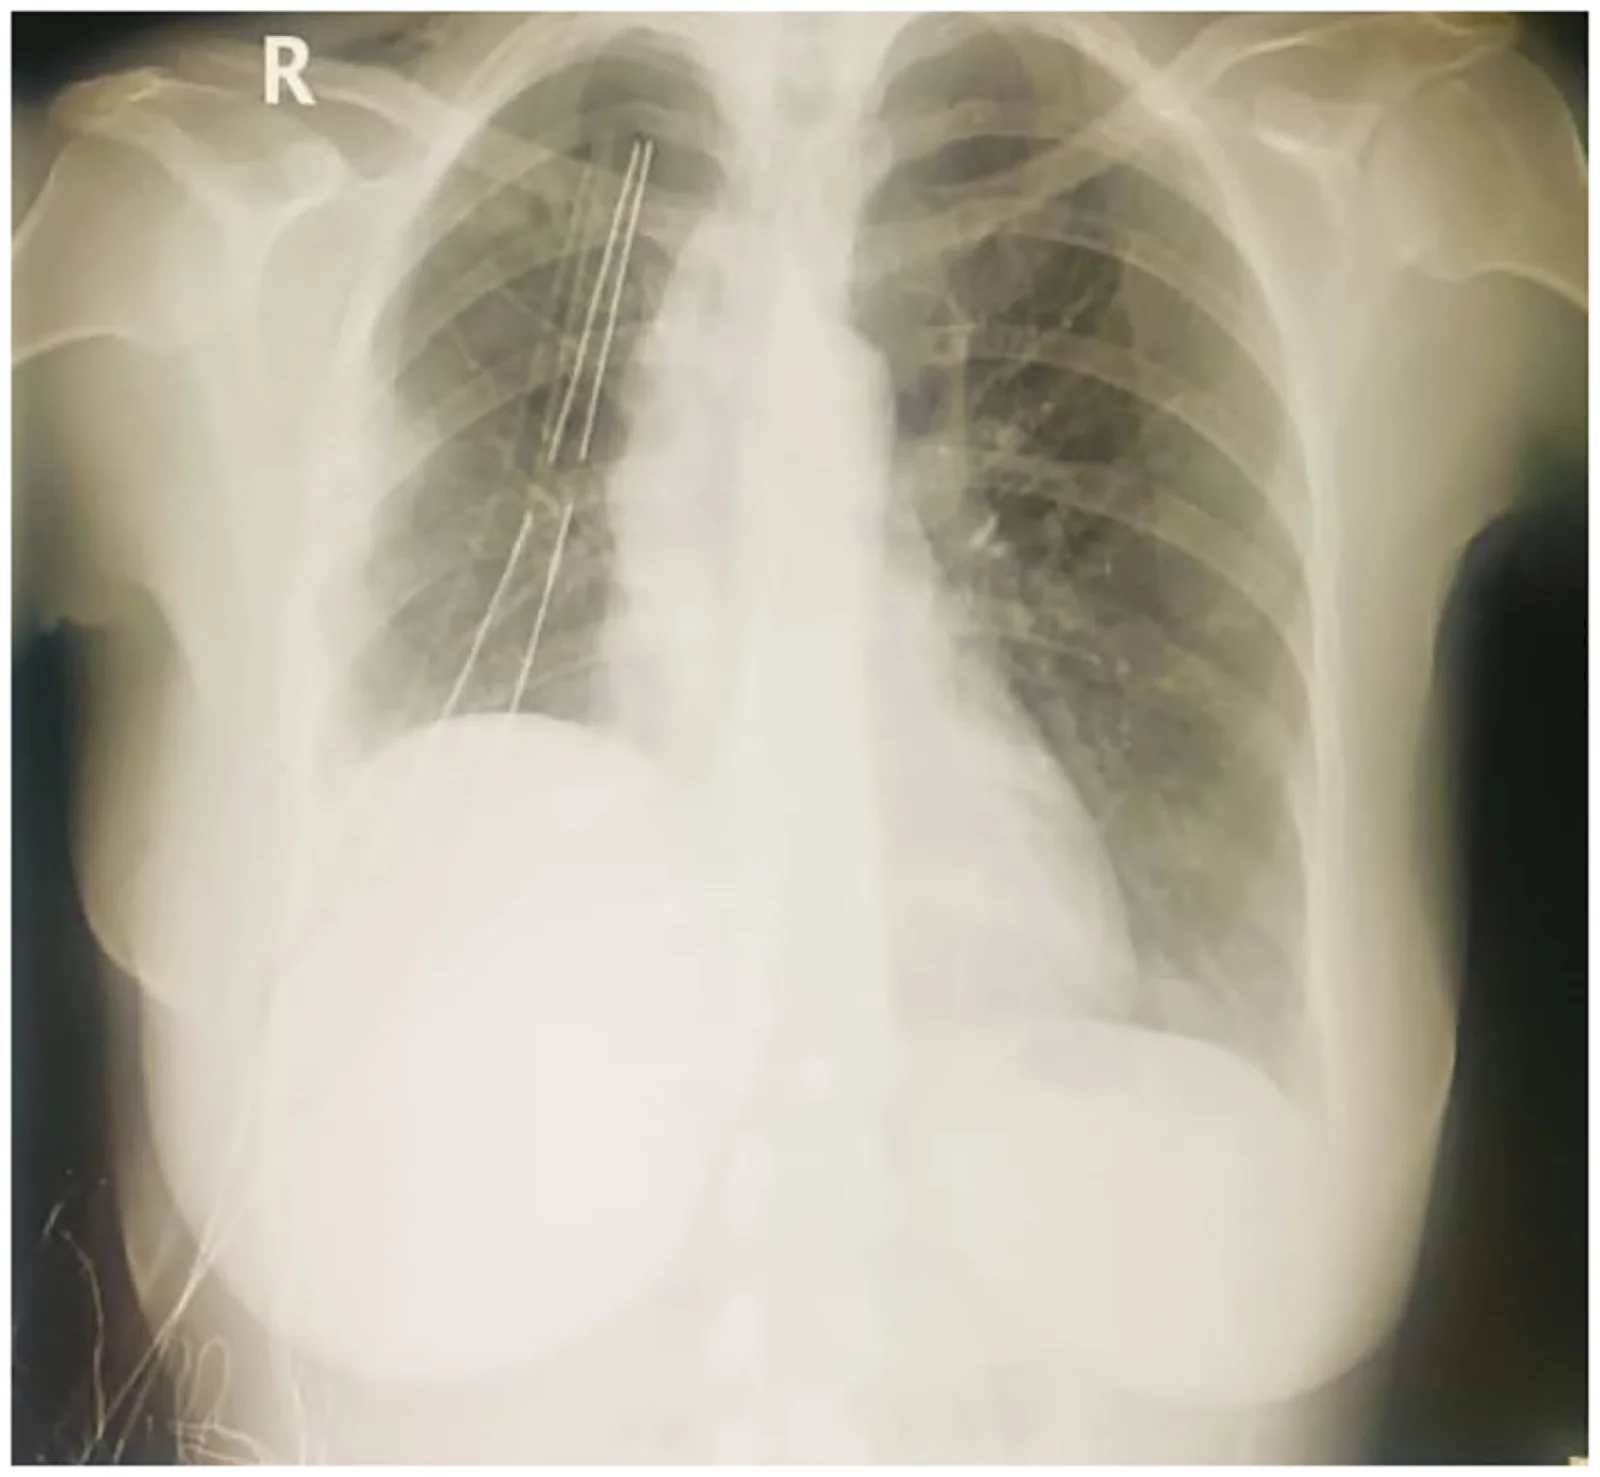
Immediate postoperative chest radiograph. Portable chest radiograph showing satisfactory re-expansion of the remaining right lung, with expected post-bilobectomy volume loss and mild rightward mediastinal shift. The chest drain is appropriately positioned, without significant pneumothorax or pleural effusion.
